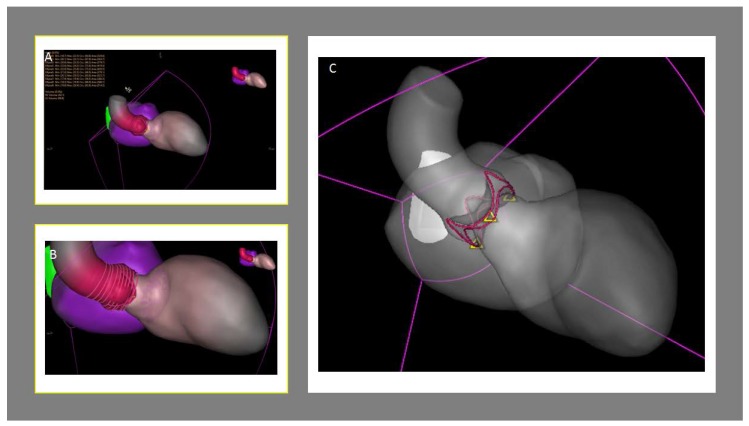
An investigational device of next-generation of real-time echocardiography-fluoroscopy fusion imaging (EchoNavigator) allows, based on a 3D echocardiographic dataset, the establishment of a 3D heart model. (A,B) The 3D heart model is automatically co-registered to the patient. Once the patient-specific 3D heart model has been generated, sizing of the aortic valve (not validated) can be exported from the system. (C) Moreover, the interventionalist can rotate the C-arm to a position where all three hinge points of the several leaflets are aligned in one plane. It can predict the optimal angulation for transcatheter aortic valve replacement (TAVR) prosthesis deployment and needs to be evaluated further.
